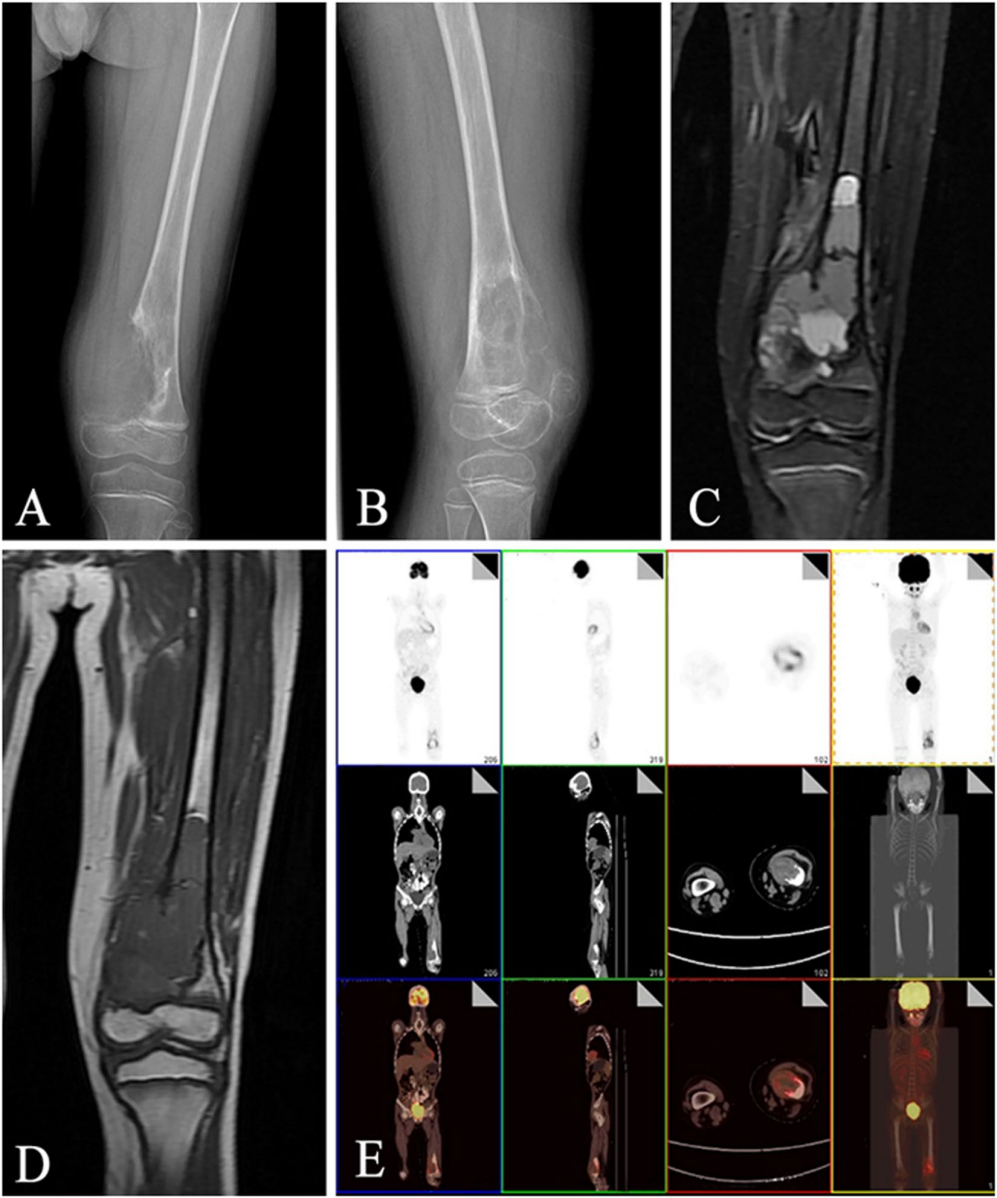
Preoperative imagological data. (A,B) Malignant tumor in the left distal femur was observed in anteroposterior (A) and lateral (B) X-ray radiographs. (C,D) Malignant tumor was confirmed from T1-weighted (C) and T2-weighted (D) magnetic resonance images. (E) Positron emission tomography-computed tomography was carried out to confirm the absence of metastasis.
